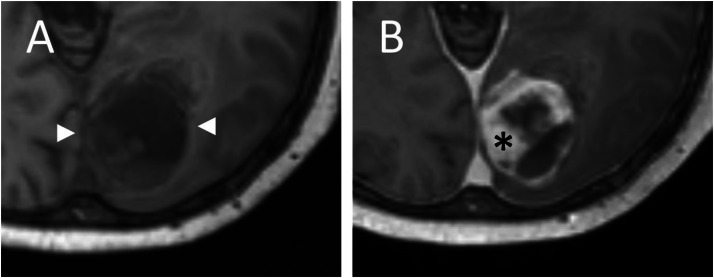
Non-contrast (A) and contrast-enhanced (B) axial T1-weighted imaging showing a heterogenous hypointense mass (arrowheads) with heterogenous enhancement (star) after contrast injection.
